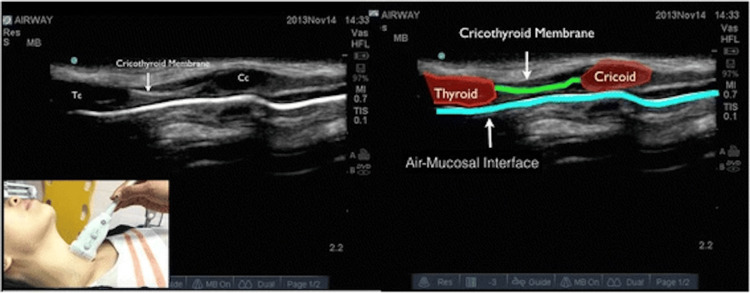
Airway ultrasound for identifying the CTM Cricoid cartilage, thyroid cartilage, and CTM in a longitudinal plane. Cc: cricoid cartilage; CTM: cricothyroid membrane; Tc: thyroid cartilage Osman and Sum (2016) [31]; Creative Commons Attribution (CC BY) license
